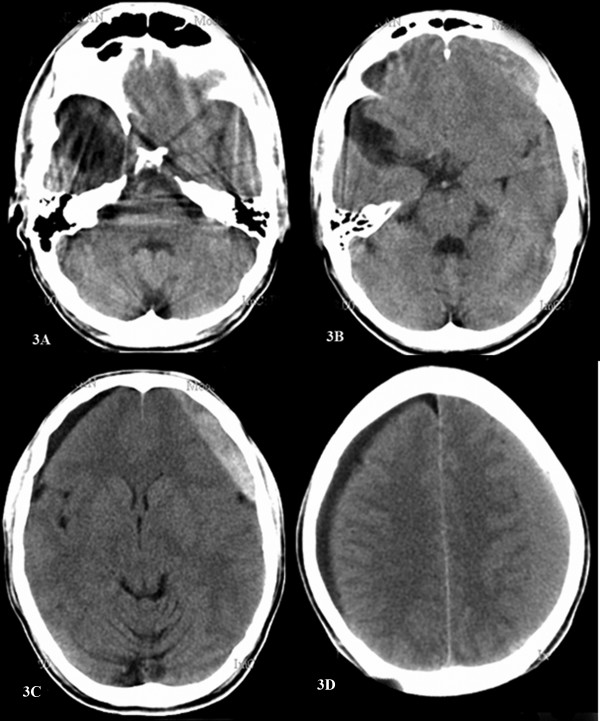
Computed tomographic scan of the head without contrast showing an arachnoid cyst in the right temporal fossa. A, B) an ipsilateral subdural hygroma and C, D) a contralateral subdural hematoma.
